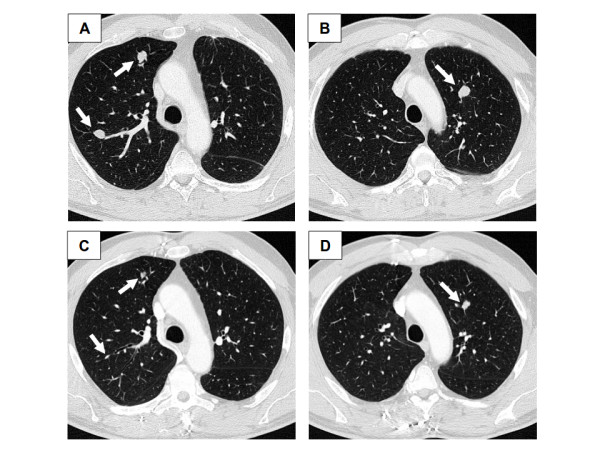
Response of lung metastases to gemcitabine-docetaxel chemotherapy. Chest CT-scan performed in October 2009 (A-B) and in August 2010 (C-D) showing partial response of lung metastases (arrows) after 9 cycles of second-line gemcitabine-docetaxel combination.
